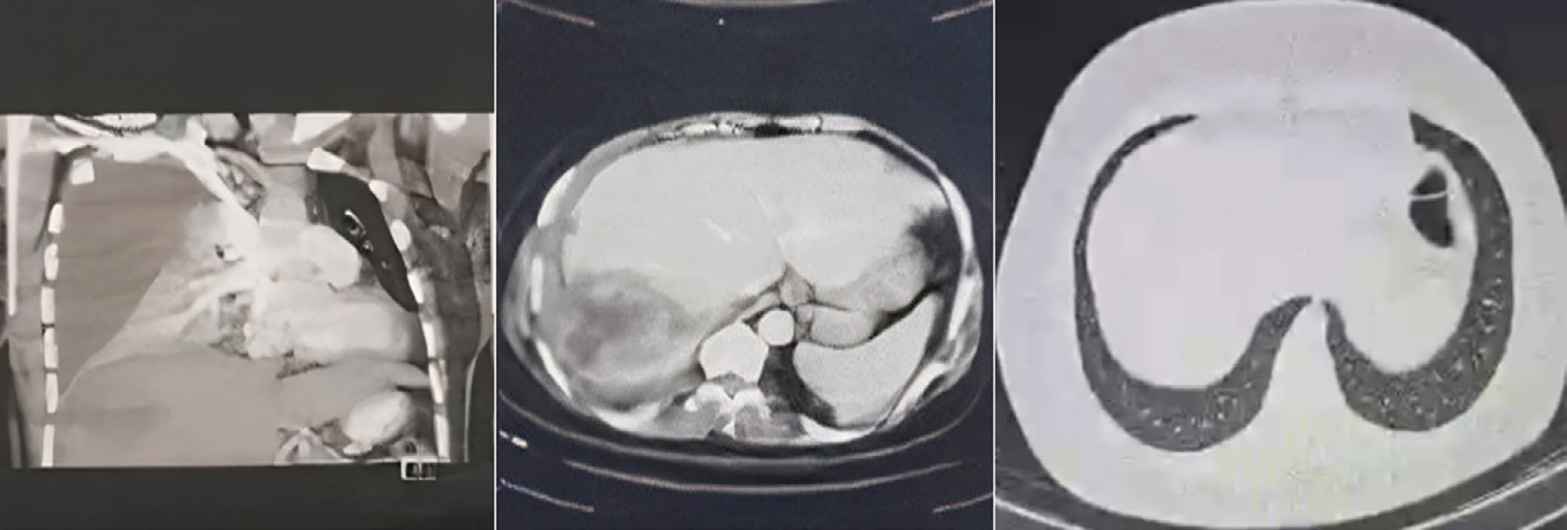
Evolution of chest CT scans from presentation (left), diagnosis (middle), to cure (right).
